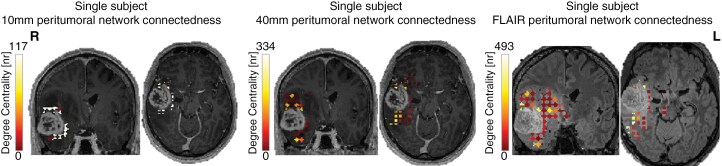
10 mm, 40 mm, and FLAIR peritumoral network connectedness. This figure visualizes peritumoral network connectedness in one single subject, contrasting the 10 mm (left panel), 40 mm (middle panel), and FLAIR-based (right panel) differences between lesional and contralesional degree centrality. Central, highly connected peritumoral nodes are indicated by a high degree centrality value and less connected nodes by a low degree centrality value.
